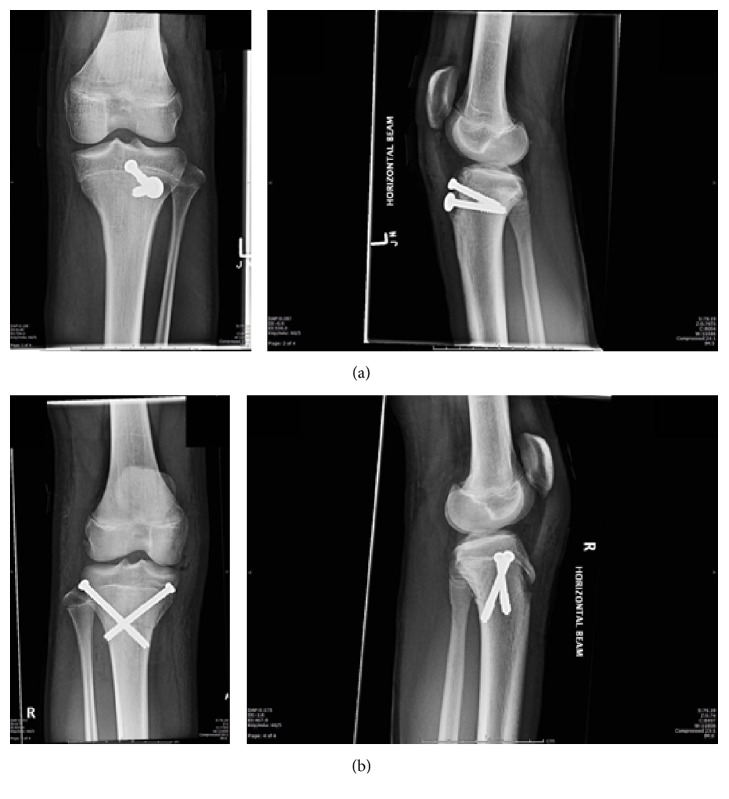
Day 1 postoperative plain radiographs of left (a) and right (b) knees following ORIF.
